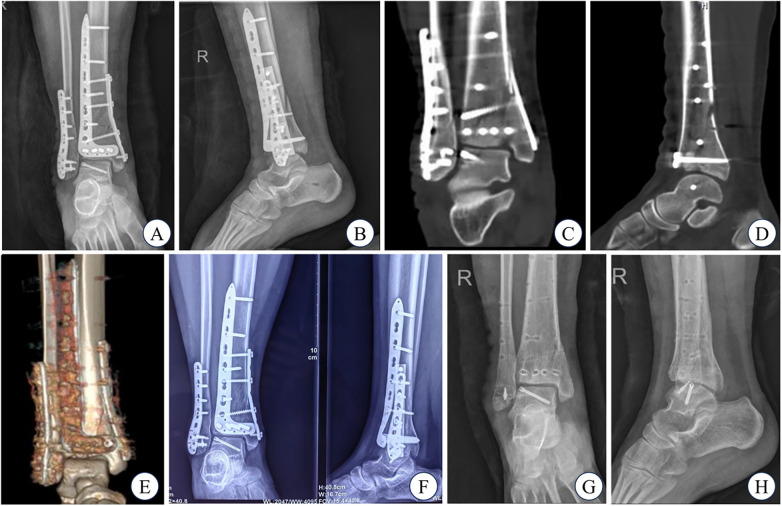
(A–E) Postoperative follow-up with ankle anteroposterior/lateral x-rays and CT 3D reconstruction demonstrated satisfactory fracture reduction with a well-aligned articular surface; (F) one-year postoperative x-rays confirmed solid fracture union without signs of traumatic arthritis; (G,H) internal fixation hardware was removed two years postoperatively.
